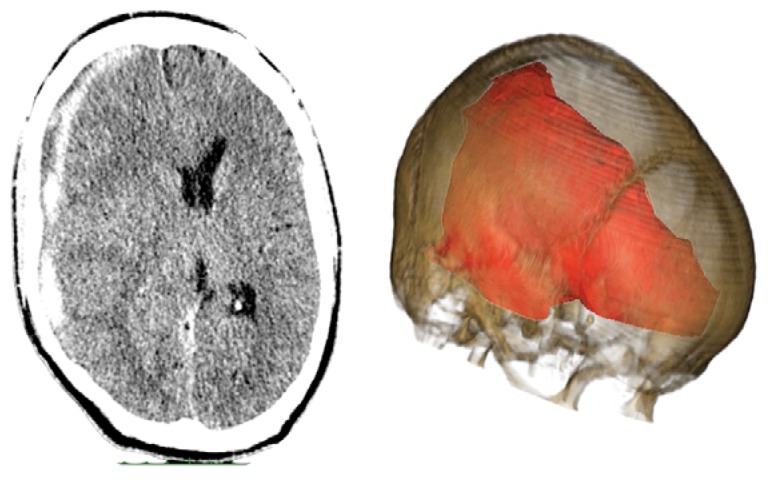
CASE 2. Preoperative CT scans. In patients with subdural hematomas without brain swelling is possible to perform an endoscopic resection. However the entry point is essential for a complete resection. The image makes it easier to calculate the position of the burr-hole so that the endoscope can be inserted adjacent to the hematoma over the entire brain convexity.
